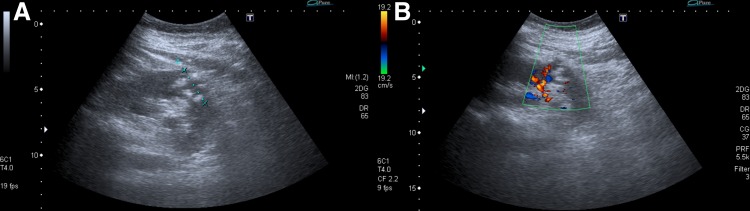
Renal abscess located dorsally and caudally from the cyst, diameter 29 mm, hypoechoic content with floating particles (A), increased perfusion around (B), by ultrasonography.
